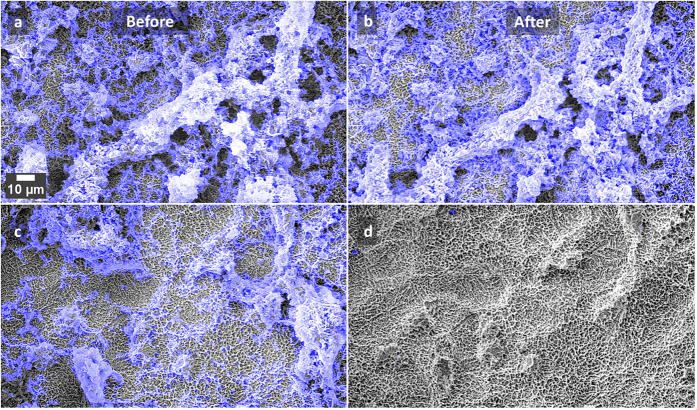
Examples of SEM images of the SLA discs, before and after treatment with the ultrasonic scaler held 1 mm away for 30 s, at low power (a,b) and medium power (c,d). The blue overlay shows the automatic detection of bacteria.
